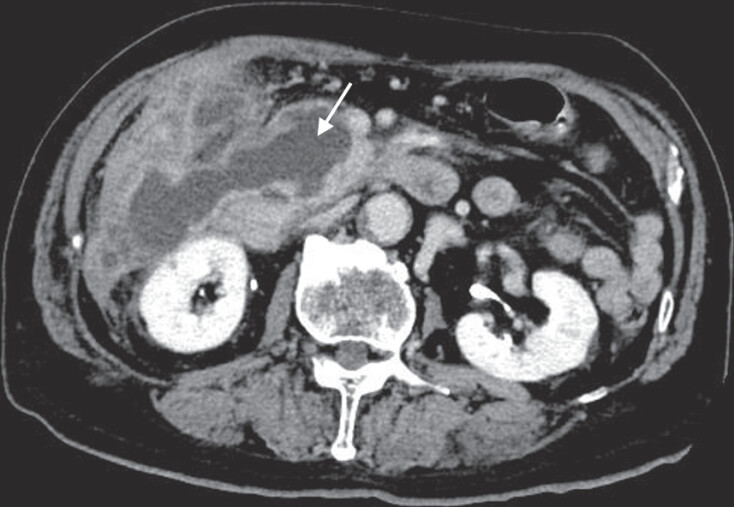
Contrast-enhanced computed tomography showing a large
